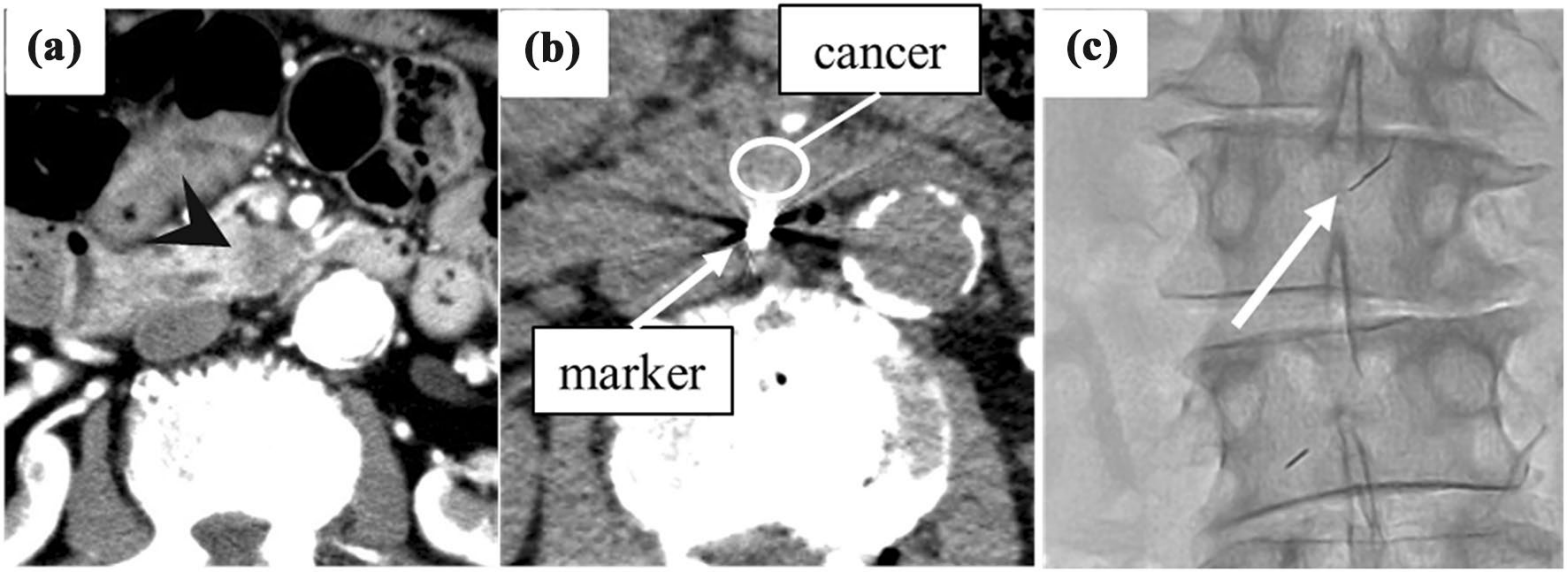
A case of tracking in parallel to the fluoroscopy beam. a Pre-procedural dynamic contrast-enhanced (CE) CT of the cancer target in the pancreas tail (white arrow). b Post-procedural plain CT shows two fiducial markers that were implanted on opposite ends of the cancer aligned with a fluoroscopy beam (white arrow), preventing the CyberKnife system from distinguishing the two separate markers
